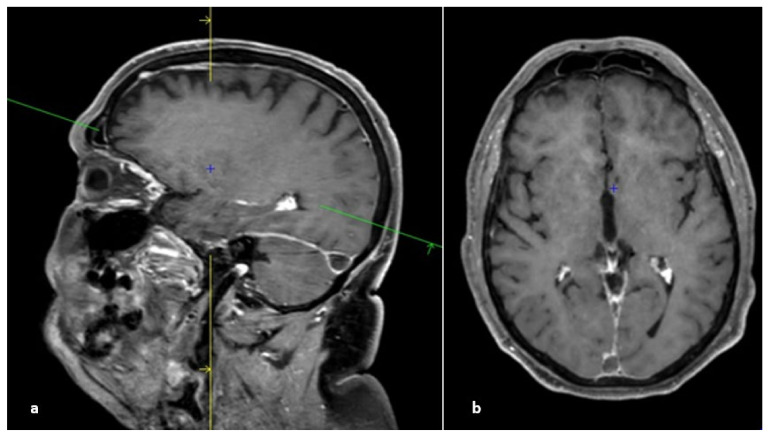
3D contrast-enhanced T1-weighted gradient-echo images. (a) Sagittal plane; “empty delta” sign in the lateral sinus. (b) Axial plane; empty delta sign in the superior sagittal sinus. The enhancement related to the injection occurs around the thrombus, which appears in relative hyposignal.
